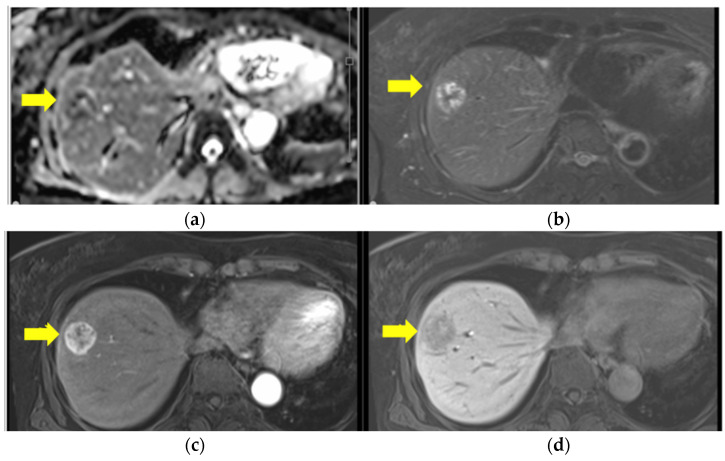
Ethoxibenzyl (EOB) magnetic resonance imaging before hepatectomy. (a) T1W1: mixed high and low, (b) T2W1: peripherally high signal. There is a well-circumscribed round lesion in S8 with ring enhancement in the EOB-arterial phase (c) and a low signal in the EOB-hepatic phase (d).
